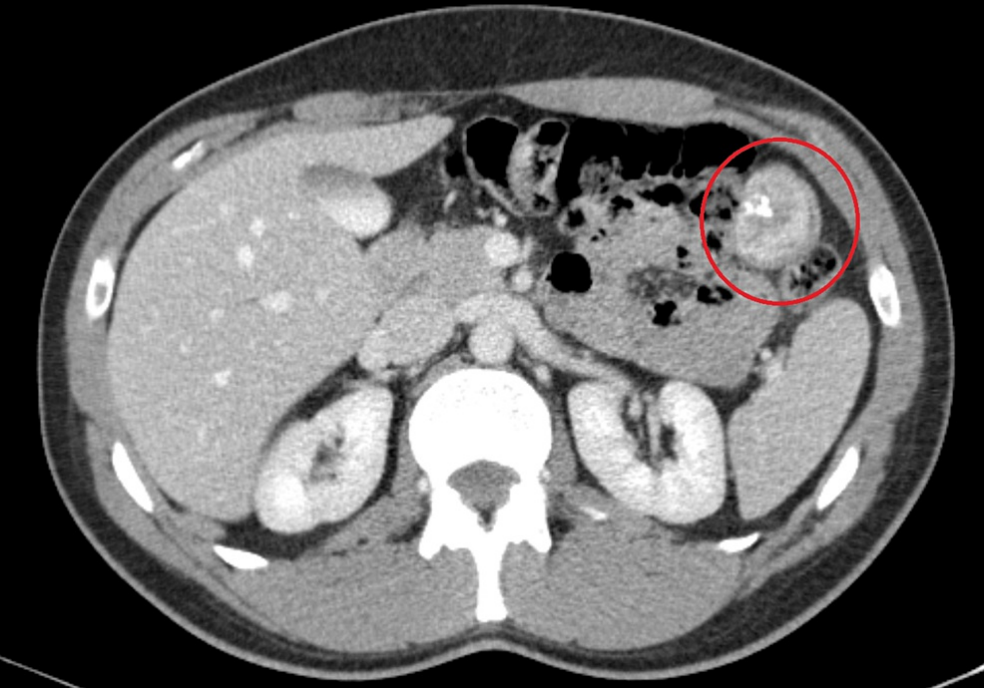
Abdominal CT scan A 3.5cm tumor on small bowel loop, with flush, is presented as a possible hemorrhage source.
